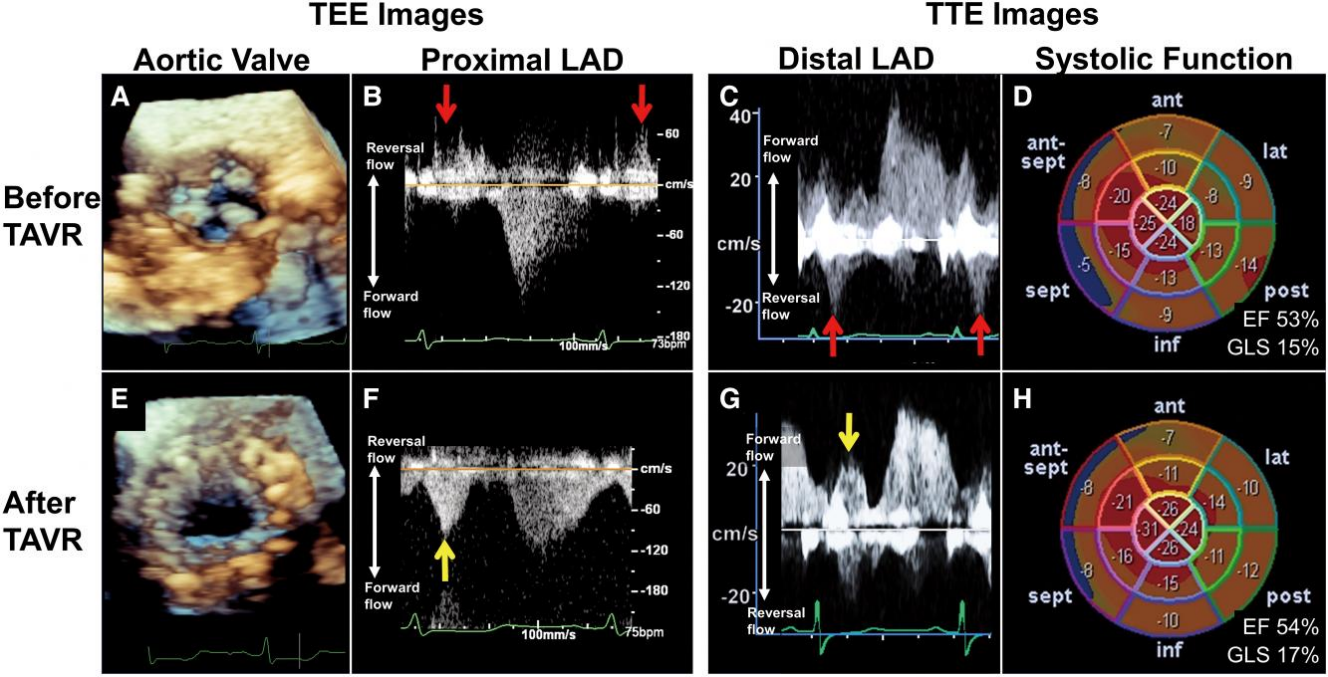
(*A*) The aortic cusp motion is severely restricted. (*B* and *C*) Transoesophageal and transthoracic echocardiography with pulse-wave Doppler scans show proximal and distal left anterior descending coronary artery flows, respectively. The left anterior descending coronary artery flow before transcatheter aortic valve replacement shows systolic coronary flow reversal (red arrows). (*D*) Transthoracic echocardiography scan before transcatheter aortic valve replacement shows a preserved left ventricular ejection fraction of 53% but a low endocardial global longitudinal strain of 15%. (*E*) A 26 mm self-expandable valve was successfully implanted, and the severe aortic stenosis was relieved. (*F* and *G*) Transoesophageal and transthoracic echocardiography scans after transcatheter aortic valve replacement show that the left anterior descending coronary artery flow in systole has changed from reverse to forward (yellow arrows). (*H*) Transthoracic echocardiography scan after transcatheter aortic valve replacement shows that endocardial global longitudinal strain has increased to 17%. EF, ejection fraction; GLS, global longitudinal strain; LAD, left anterior descending coronary artery; TAVR, transcatheter aortic valve replacement; TEE, transoesophageal echocardiography; TTE, transthoracic echocardiography.
